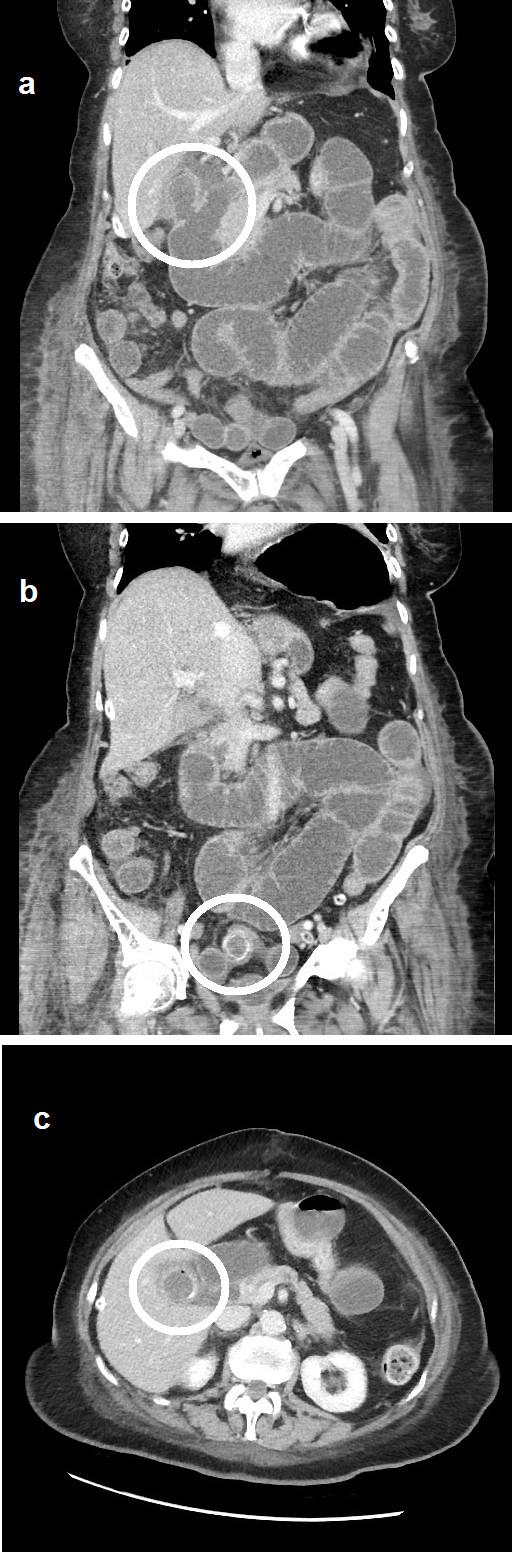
Computed tomography scan performed after the initial laparotomy. (a) Coronal computed tomography image of a cholecysto-duodenal fistula. (b) Coronal computed tomography image of small bowel calculus with proximal dilatation. (c) Axial computed tomography image of an additional calculus in the gallbladder.
